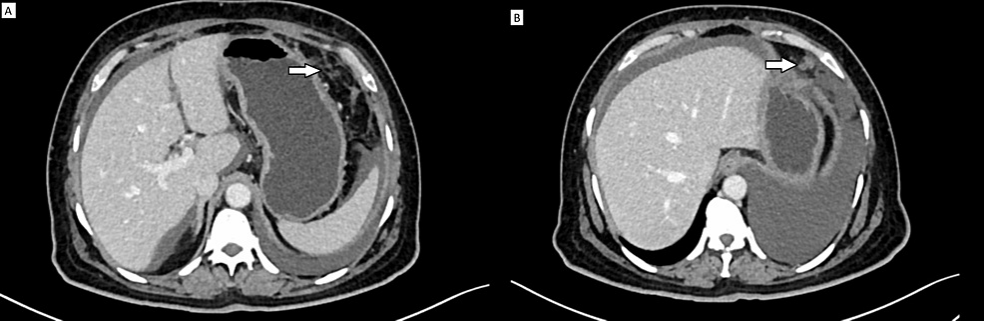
CT scan images of gliomatosis peritonei (Source: own photo) Post-contrast computed tomography (CT) axial section shows omental and mesenteric deposits in form of A) subtle fat stranding (arrow) and B) nodule (arrow)
